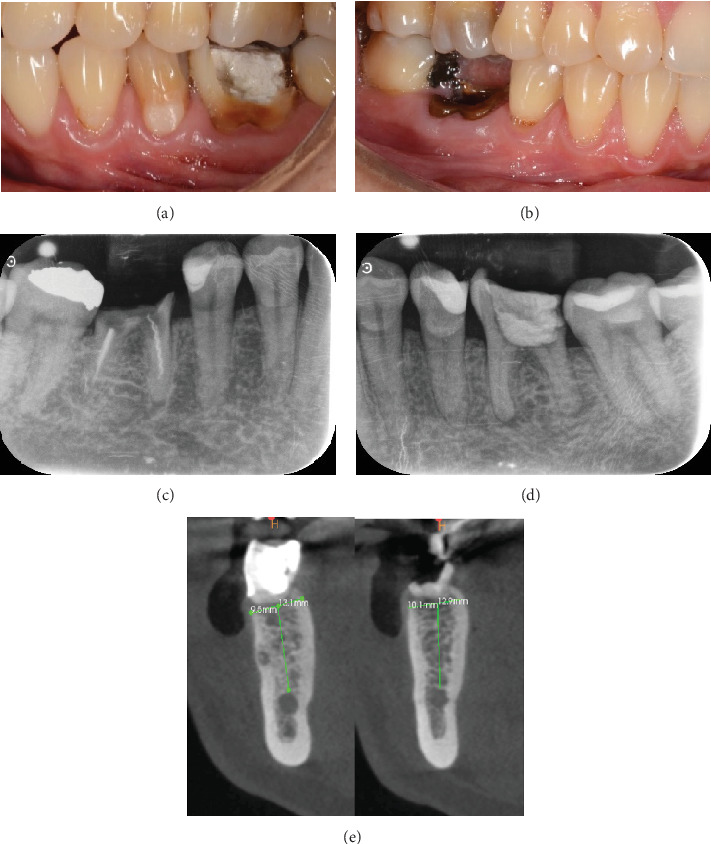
Preoperative situation: (a) clinical situation of Tooth 19, (b) clinical situation of Tooth 30, (c) initial periapical x-ray of Tooth 30, (d) initial periapical x-ray of Tooth 19, and (e) CBCT area of Teeth 19 and 30, respectively.
